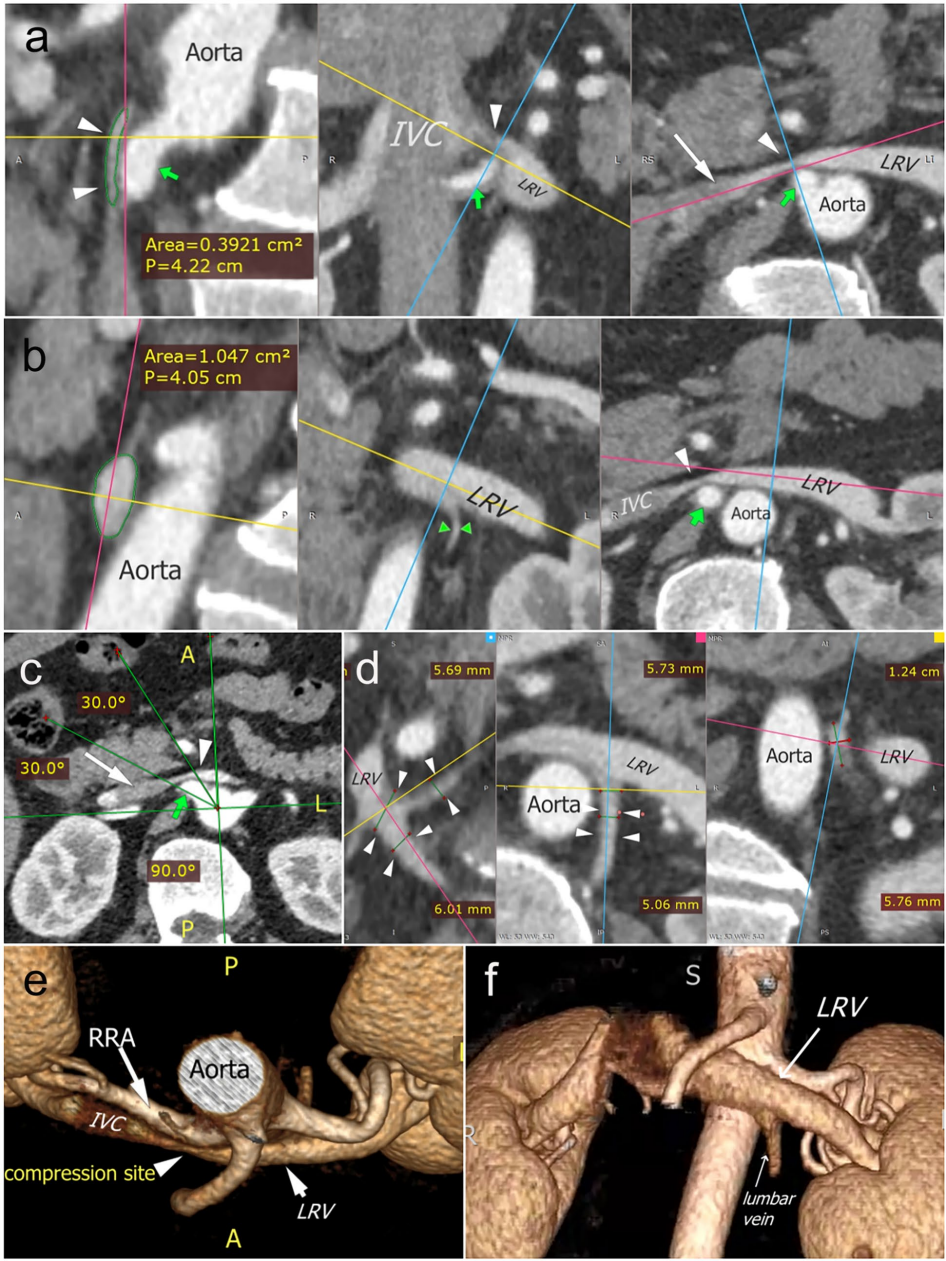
CT imaging showed a notable LRV compression by RRA in a 58-year-old male with membranous nephropathy. The ending segment of LRV was compressed by the proximal RRA (green arrows in a–c), arising at a 10:30 clockwise direction (c). The narrowest site was at the compression site (white arrowheads in a–c and e), and the maximum-to-minimum area ratio was calculated as 2.93 depending on the measurements on a and b (circled green lines). The dilated lumber veins could be recognized (d, f). IVC, inferior vena cava; LRV, left renal vein; RRA, right renal artery; CT, computed tomography. Long-shafted white arrows (without text), IVC; paired arrowheads, collateral veins
